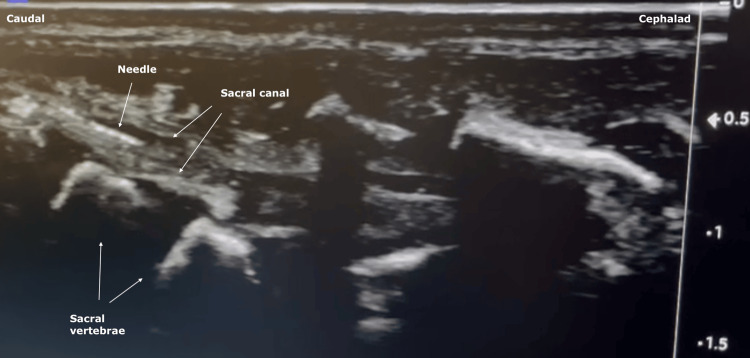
Ultrasound view of the longitudinal plane
